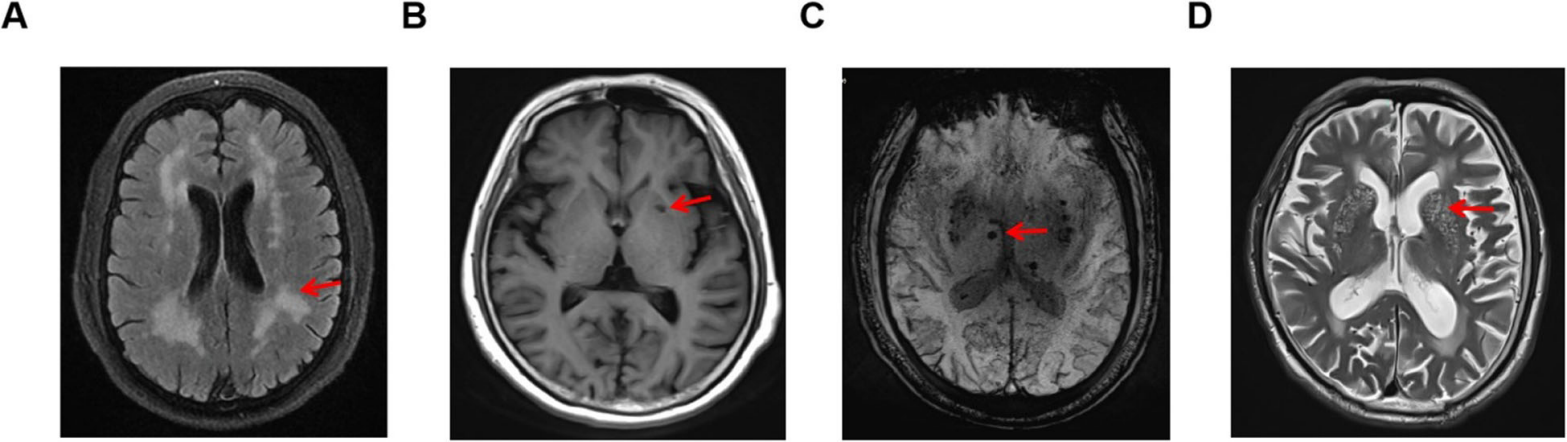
MRI manifestations of cerebral small vessel disease. a WMH, high signal lesions on fluid-attenuated inversion recovery sequence; b Lacunes, small cerebrospinal fluid-containing cavities larger than 3 mm and smaller than 15 mm in diameter on T1-weighted; c CMB, small punctuate areas of hypointensity on susceptibility-weighted imaging measuring up to 10 mm in diameter; d PVS, the small deep perforating arterioles as they pass through the deep grey and white matter, made visible on T2-weighted by containing increased fluid of similar signal to CSF
